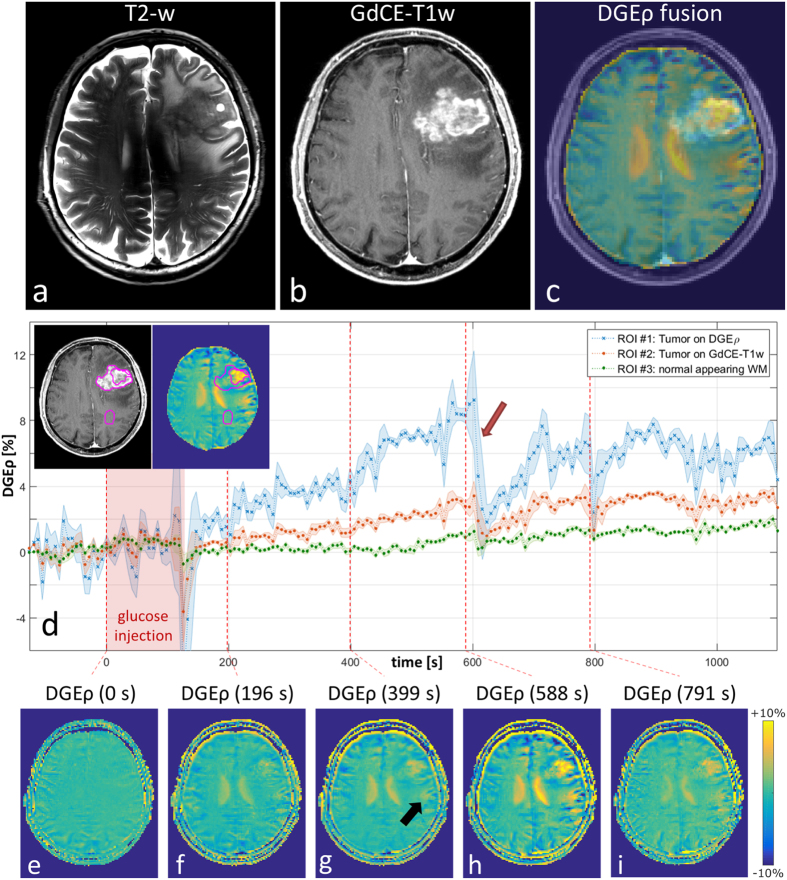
Accelerated and quantitative T_1ρ_-weighted dynamic glucose enhanced MRI applied in the study of a patient with a glioblastoma at B_0_ = 7T. (a) T_2_-weighted image acquired at 7T, (b) gadolinium-enhanced T_1_-weighted (GdCE-T1w) image acquired at 3T, (c) fusion of the GdCE-T1w image and the T_1ρ_-weighted dynamic glucose enhancement (DGE_ρ_) obtained at t = 588 s. (d) Unsmoothed DGE_ρ_ time curves with a temporal resolution of less than 7 seconds in a tumor-ROI selected on DGE_ρ_ (ROI #1), a second tumor-ROI selected on the GdCE-T1w image (ROI #2), and a ROI in normal appearing white matter (ROI #3). The error is given by the standard deviation of 5 consecutive data points and the ROIs are marked in the GdCE-T1w and DGE_ρ_ image shown in the top left corner. Increasing DGE_ρ_ values are obtained in both tumor-ROIs after the end of the glucose injection. The red arrow marks an abrupt signal drop induced by patient motion. (e–i) DGE_ρ_ images (average of 5 consecutive images) at different time points after glucose injection. Note the hyperintense region at the bottom of the tumor area (black arrow; (g)), which is not visible in the GdCE-T1w image (b).
